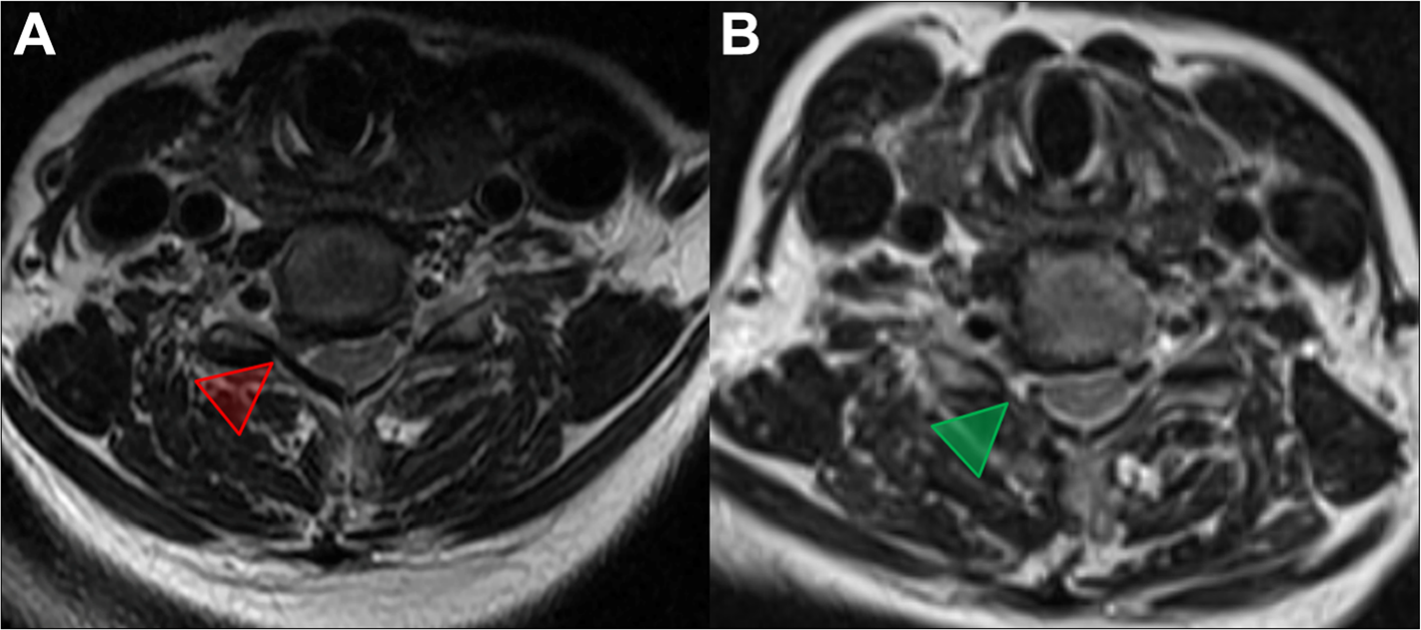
Pre- and postoperative C6-C7 MRI in axial view. a Red arrow demonstrates C6-C7 cervical disc herniation; b Green arrow indicates that C7 nerve root compression was removed
